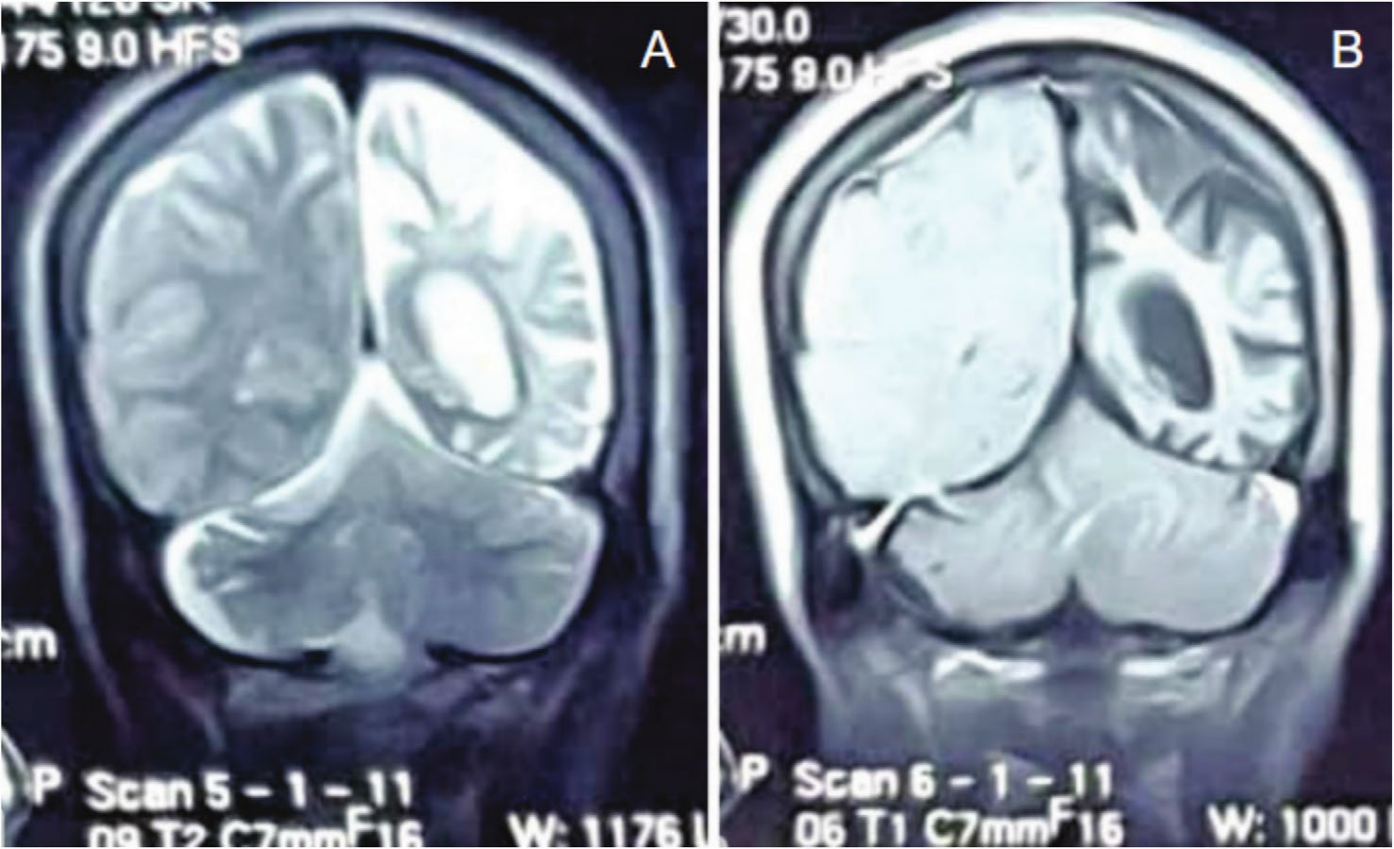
(A) Coronal T2‐weighted MRI showing left hemicerebral atrophy with ex‐vacuo dilatation of the left lateral ventricle. (B) Coronal T1‐weighted post‐contrast MRI displaying similar findings with no abnormal enhancement.
